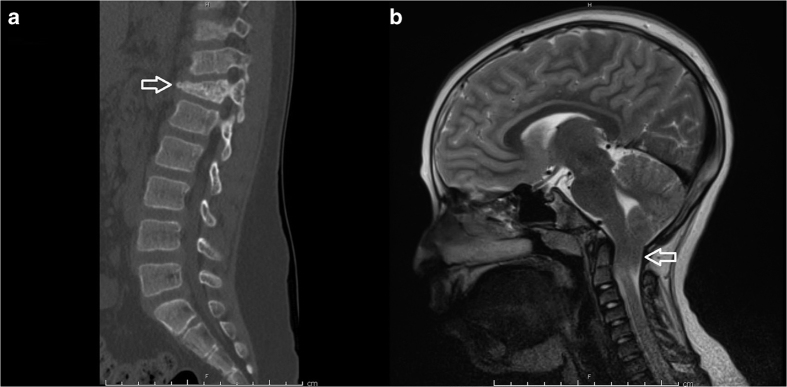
(a) Sagittal spine computed tomography image demonstrating a T12 compression fracture (arrow) that occurred after low-impact trauma in the setting of severe osteopenia. (b) Sagittal T2-weighted magnetic resonance image demonstrating significant (1.8 cm) herniation of the cerebellar tonsils (arrow) beyond the level of the foramen magnum, hallmark of a Chiari type 1 malformation. This was associated with syringohydromyelia extending through the length of the cervical and thoracic cord, the largest diameter of which measured ~3 mm at the level of C6 and C7.
